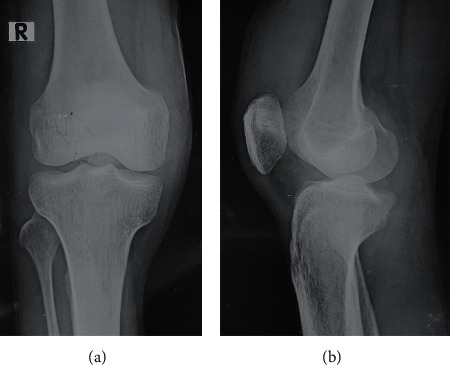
Plain radiograph of right knee joint: anteroposterior view (a); lateral view (b).
